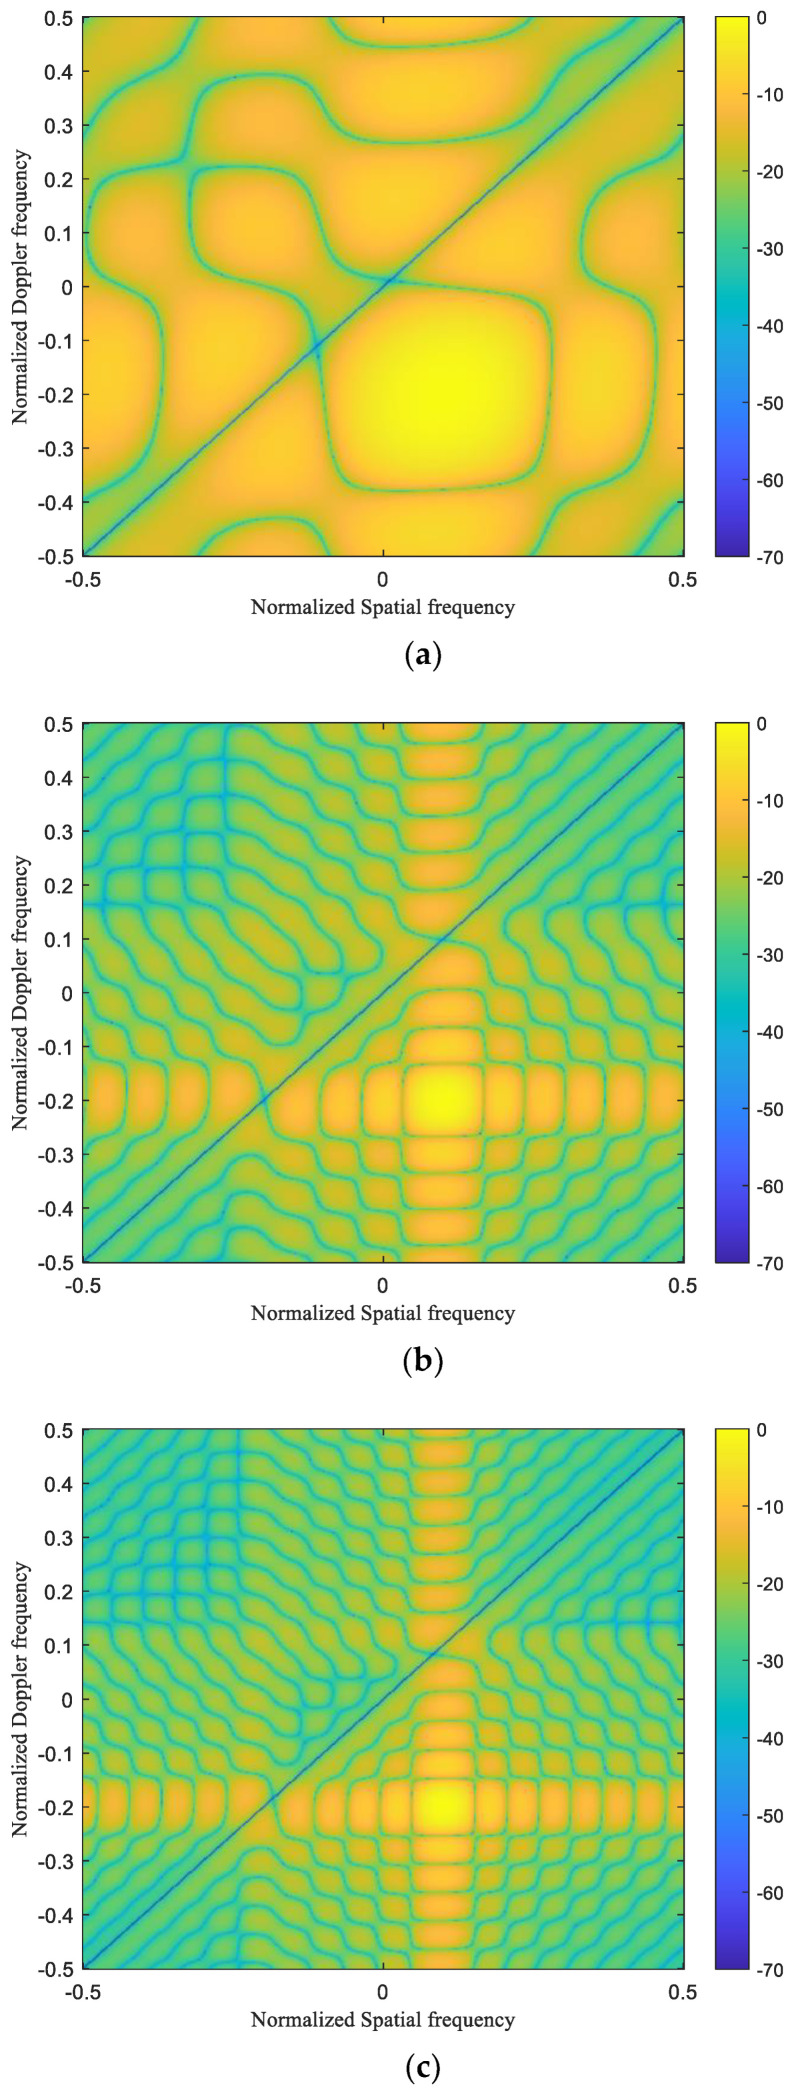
Beampatterns in angle Doppler: (a) T-STAP; (b) C-STAP; (c) TNNM-FIC-STAP.
